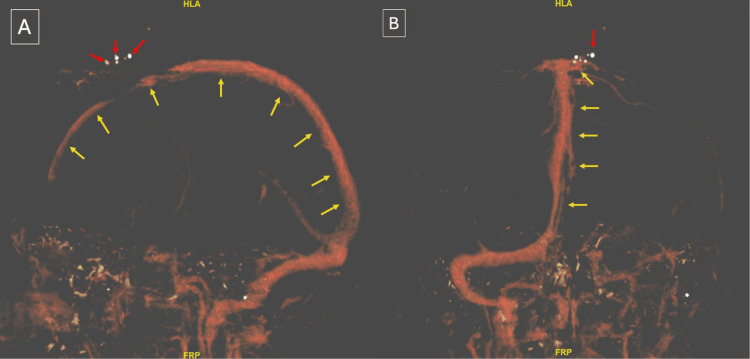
Preoperative head CT venography demonstrating the SSS (yellow arrows) and bullet fragments (red arrows). (A) Anteroposterior view. (B) Caudocranial view CT: computed tomography, SSS: superior sagittal sinus
